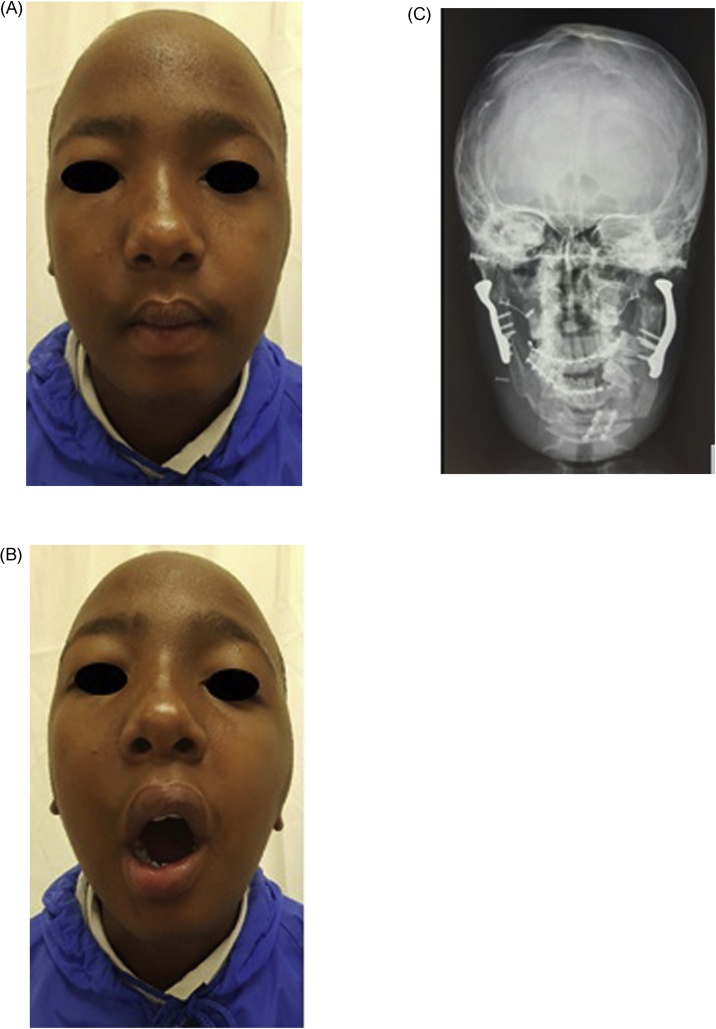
(A) Post-operative images depicting good facial symmetry. (B) Good maximum mouth opening. (C) Radiograph showing alloplastic joint prostheses.
